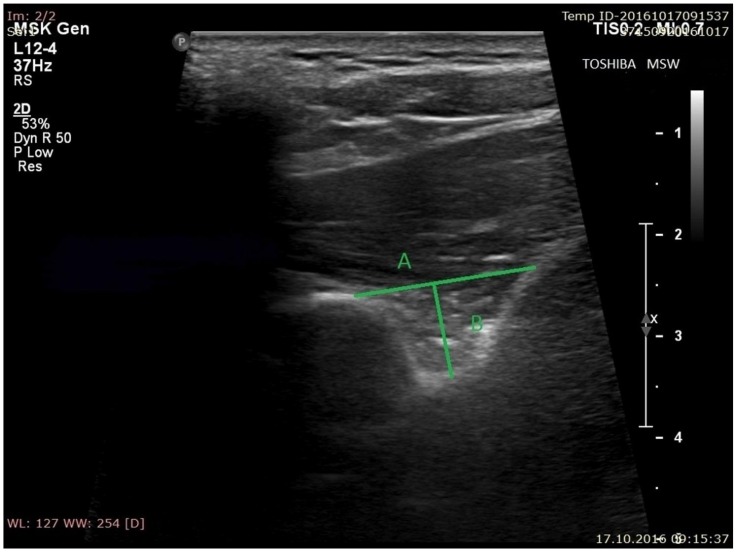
Measurements of suprascapular notch during ultrasonographic investigation. (A) the superior transverse diameter (STD); (B) the maximal depth (MD).
